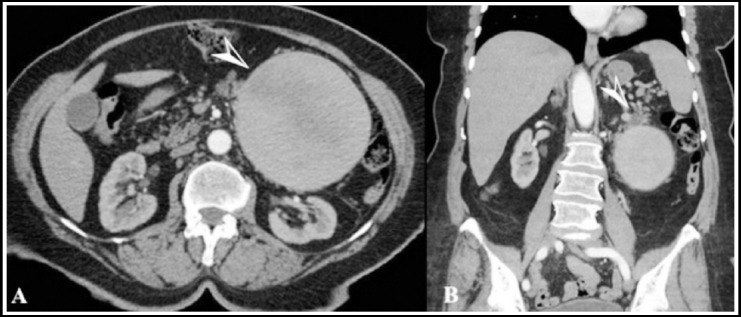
A computed tomographic (CT) angiogram; arrow head showed an aneurysm arising from the middle portion of splenic artery and arrow head showed an hypoechoic lesion arising from the distal pancreas.
